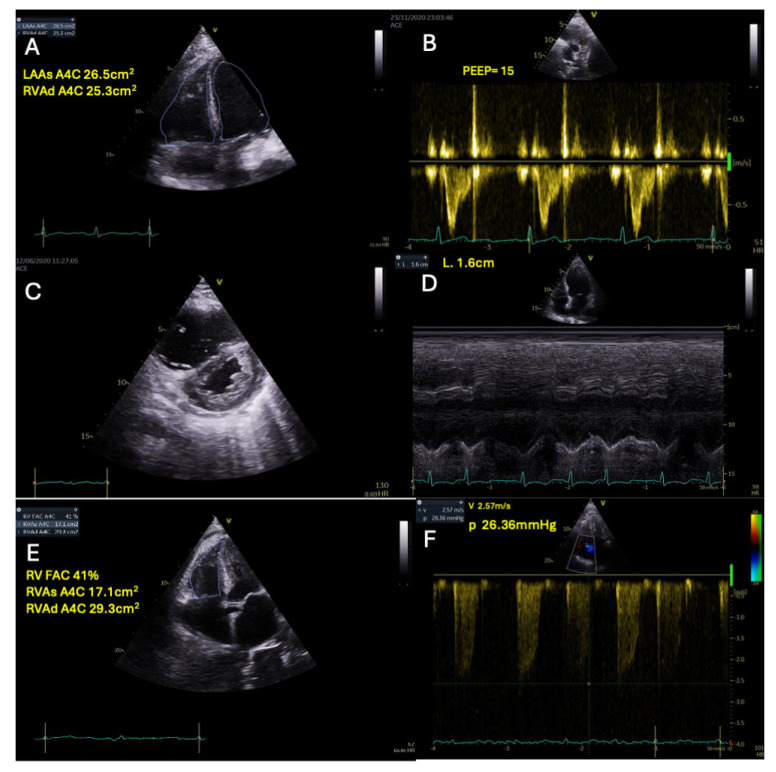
Indices of RV function. (A). Assessment of RV size through the evaluation of RV end diastolic area) (RVEDA) to LVEDA. (B) RV outflow tract velocity Time Integral (RVOT VTI). The ascending part of the RVOT VTI envelop presents a midsystolic notch which is indicative of increased pulmonary vascular resistances (PVRs). (C). D shape of the LV at the parasternal short axis indicating Acute Core Pulmonale (ACP) due to increased RV pressures. In order to conclude that ACP is due to sepsis (SCM) it is essential to exclude all other features that result to RV dusfunction. (D). Tricuspid annular Plane Systolic Excursion (TAPSE) which is borderline. (E). Fractional Area Change quantification through the equation [RVED area − RVESA)/RVESA]. (F). Estimation of RV Systolic pressure through the velocity of the envelope of tricuspid regurgitation. Using the Bernouli equation. Using the above measurements, TAPSE/PASP, a derived variable indicating right ventriculoarterial; coupling, can be evaluated. Moreover, PASP/VTI RVOT can indicate the value of PVRs.
